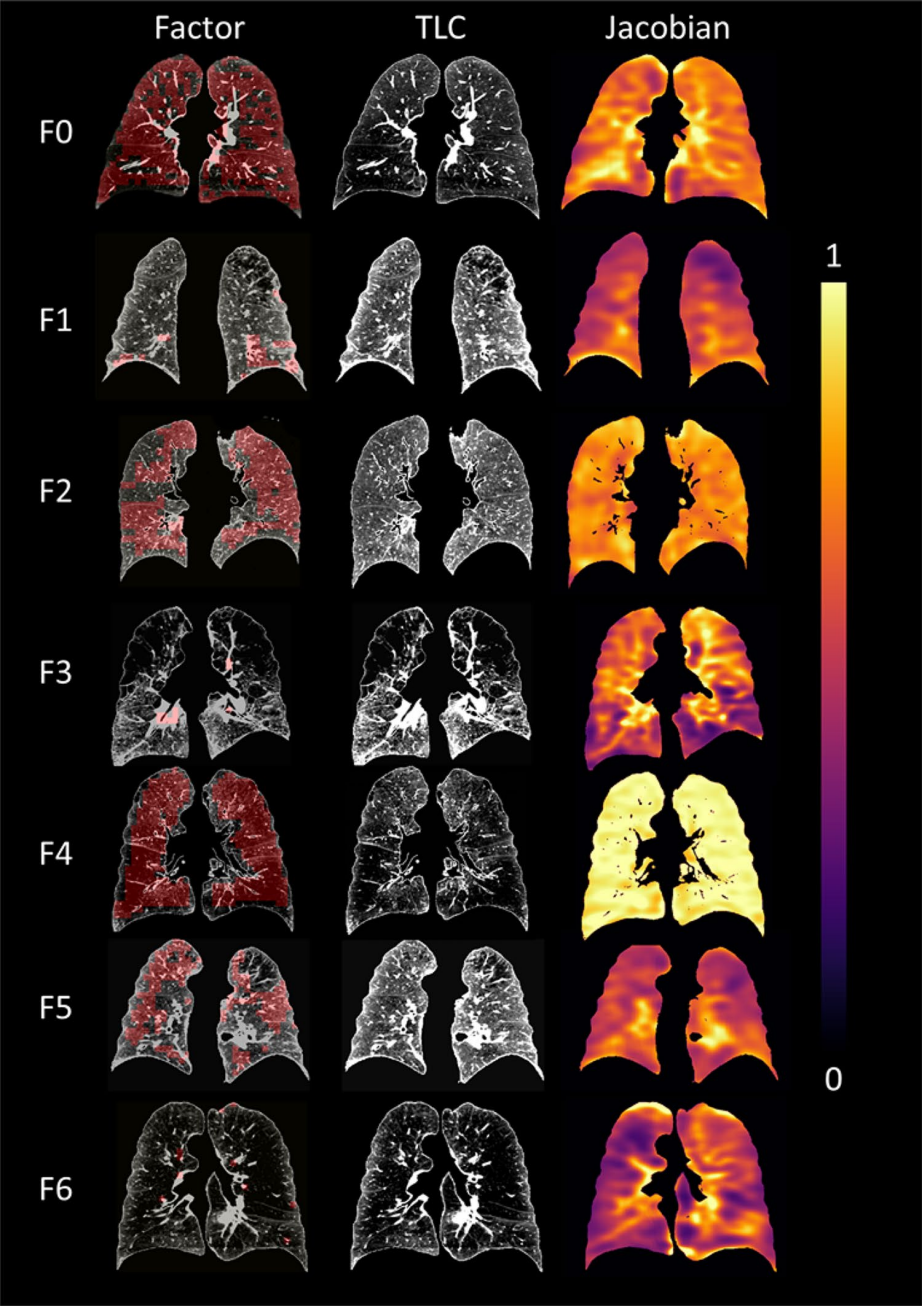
Factor-representative subjects (i.e. 99th percentile of the factor score among the subjects), shown in a coronal view (posterior–anterior). The pattern-clusters with strong contribution to each factor, ranging from F0 to F6, are shaded with red on the images in the left column. TLC images with CT intensities are displayed in the middle. Jacobian images are shown in the right column.
